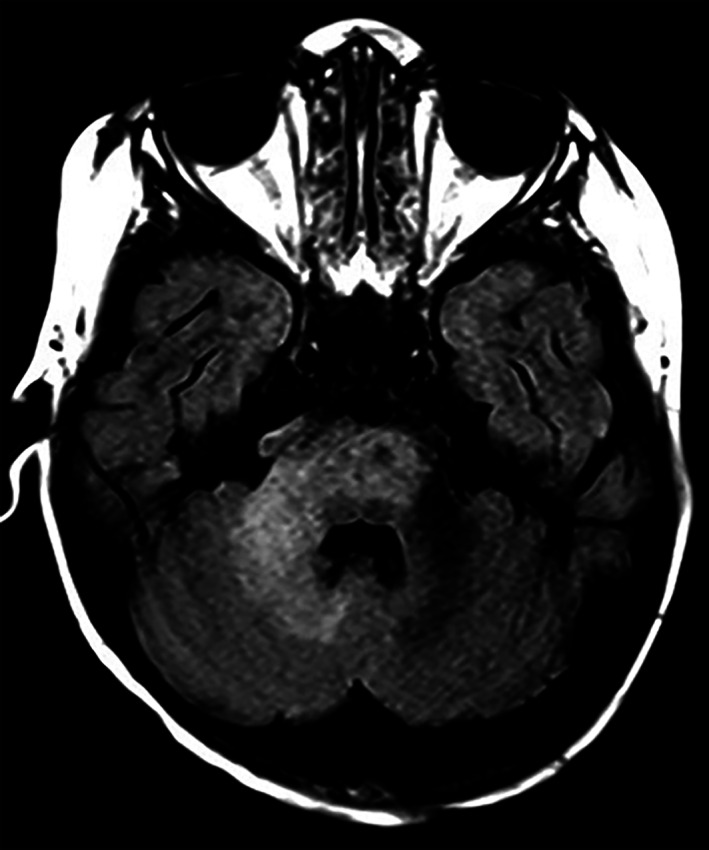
FLAIR MRI appearance. A mildly expansile T2 signal abnormality within the bilateral pons is present with extension into the right cerebellar white matter and medulla with sparing of the gray matter. No associated enhancement or diffusion restriction was present.
